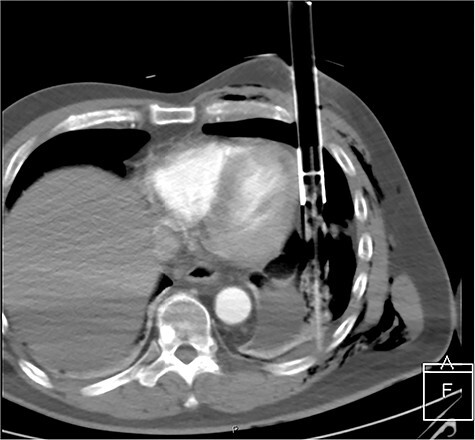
CT axial view of penetrating solar powered garden light demonstrating proximity of metal shaft to heart and the plastic tip resting against the posterior thorax.
